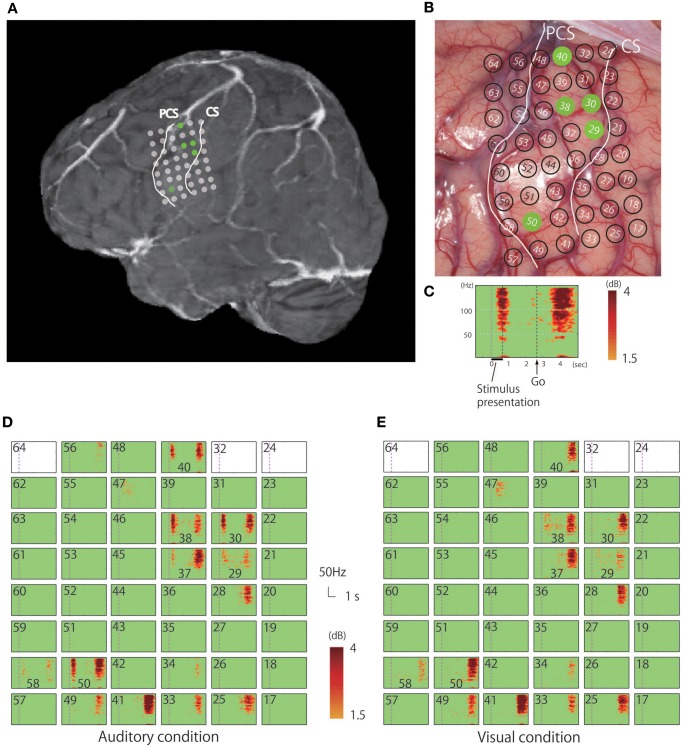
(A) Rendered MR image in which the dots indicate the positions of the electrodes from which responses to the verb generation task were recorded. (B) Positioning of electrodes overlaid on the cortical surface prior to tumor resection; green dots indicate electrodes in the precentral gyrus that recorded auditory responses. (C) Magnified view of a representative time-frequency plot (channel 50). The horizontal axis represents time, the vertical axis represents frequency, and the colors indicate the magnitudes of the response (dB) relative to the baseline period; the purple dotted line indicates the time (0 s) when the noun was presented. (D) Time-frequency plots of the recorded signal in response to the verb generation task with auditory noun presentation. (E) Time-frequency plots of the recorded signal in response to the verb generation task with visual noun presentation. Blank rectangles indicate bad channels.
